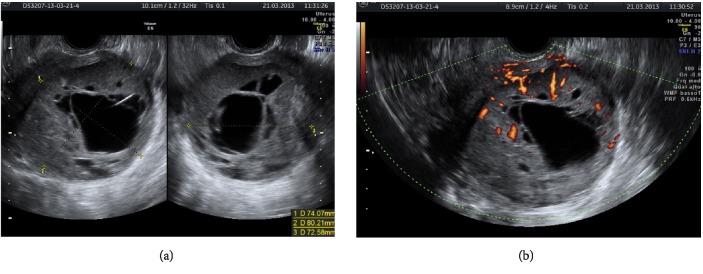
(a) M-Mode findings: enlarged uterus with inhomogeneous echogenicity. (b) Power Doppler findings: multiple anechoic areas with central and peripheral vascularization.
